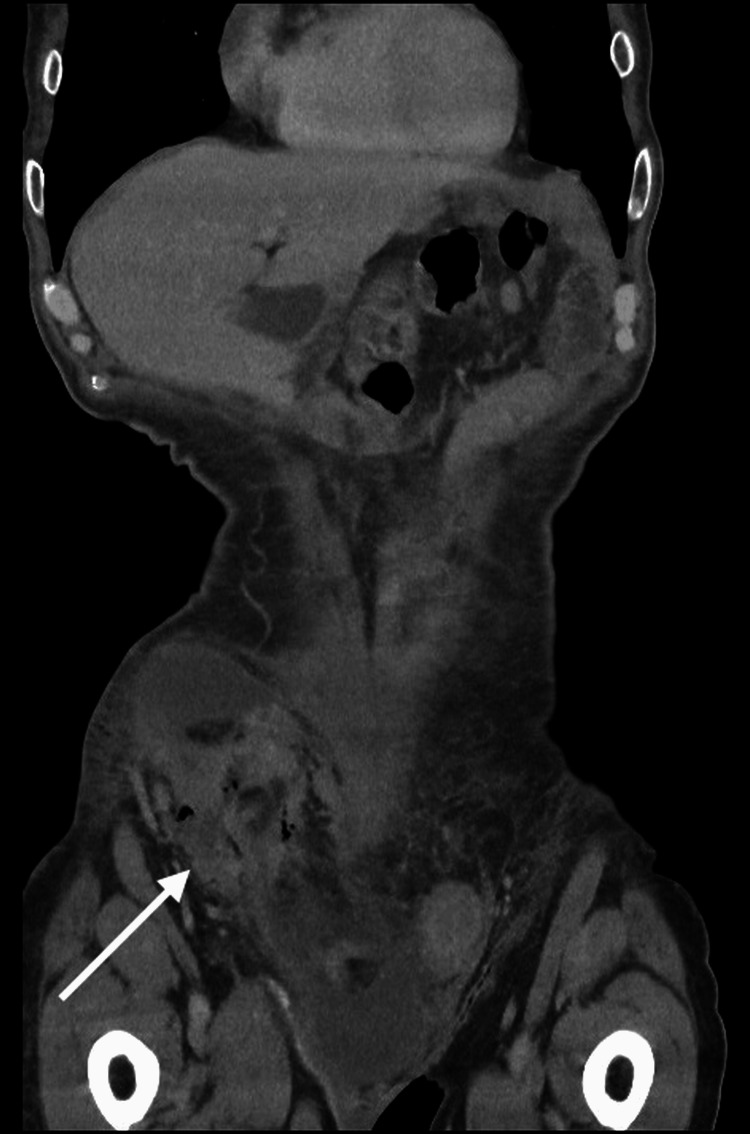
CTAP coronal plane - 1 Collection suggestive of colon perforation CTAP: computed tomography of the abdomen and pelvis
